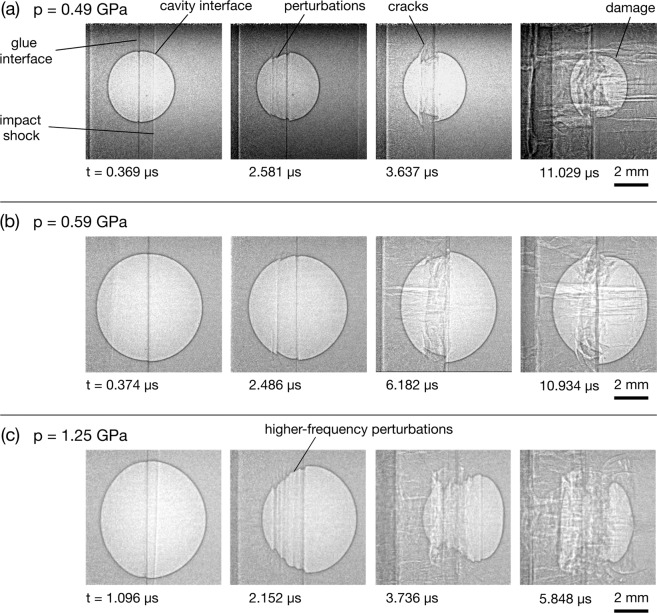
Radiograph sequences showing shock-cavity interactions in the strength-dominated regime. (a) A 4 mm cavity and 0.49 GPa shock. (b) A 6 mm cavity and 0.59 GPa shock. (c) A 6 mm cavity and 1.25 GPa shock.
